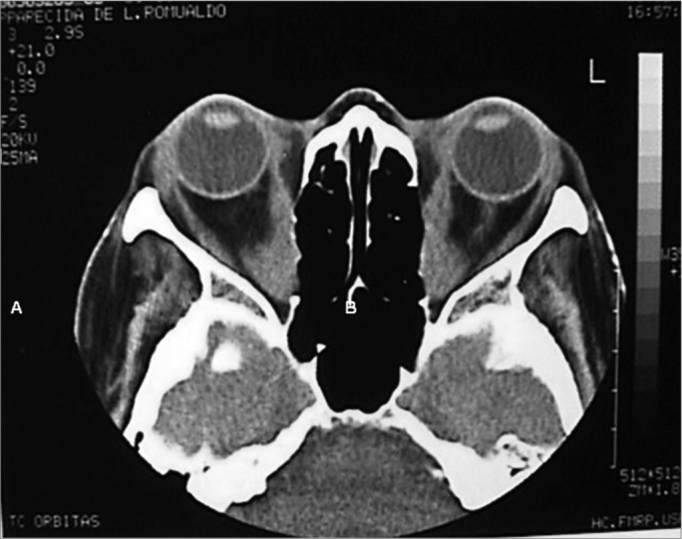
CT scans, axial views (A) of a patient with Graves’ ophthalmopathy, showing the hypertrophy of the extrinsic ocular muscles.
